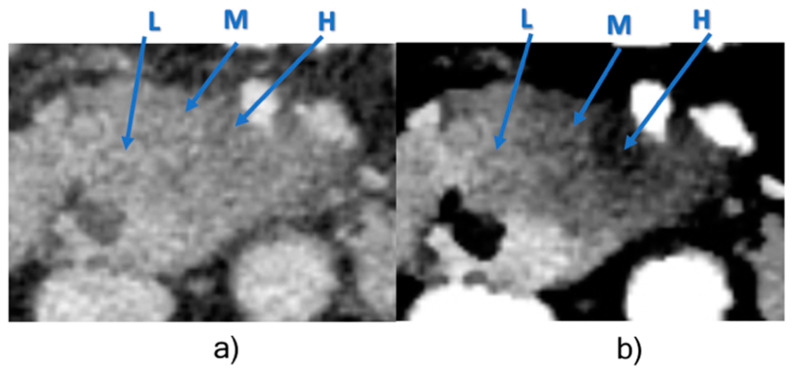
Low (L), intermediate (M) and high (H) cellularity levels within one tumor slice. (a) Conventional image. (b) monoE 40 keV image. Window settings (level/width): 50/350 for conventional CT, 250/350 for monoE 40 keV.
